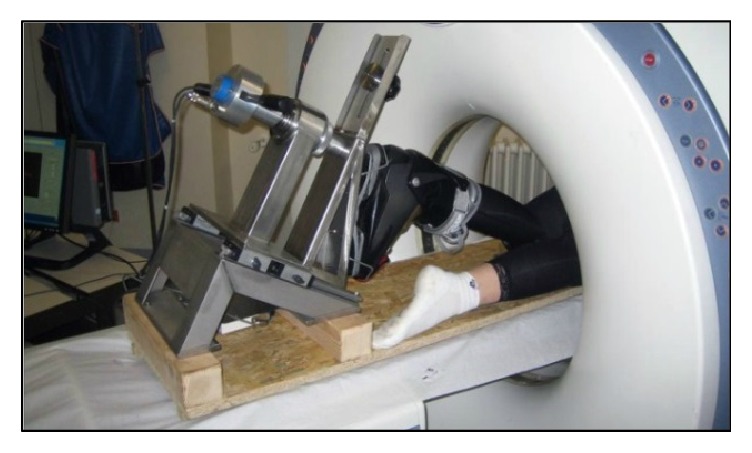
CT scanner with a patient attached to the adapted Rotameter P2.
